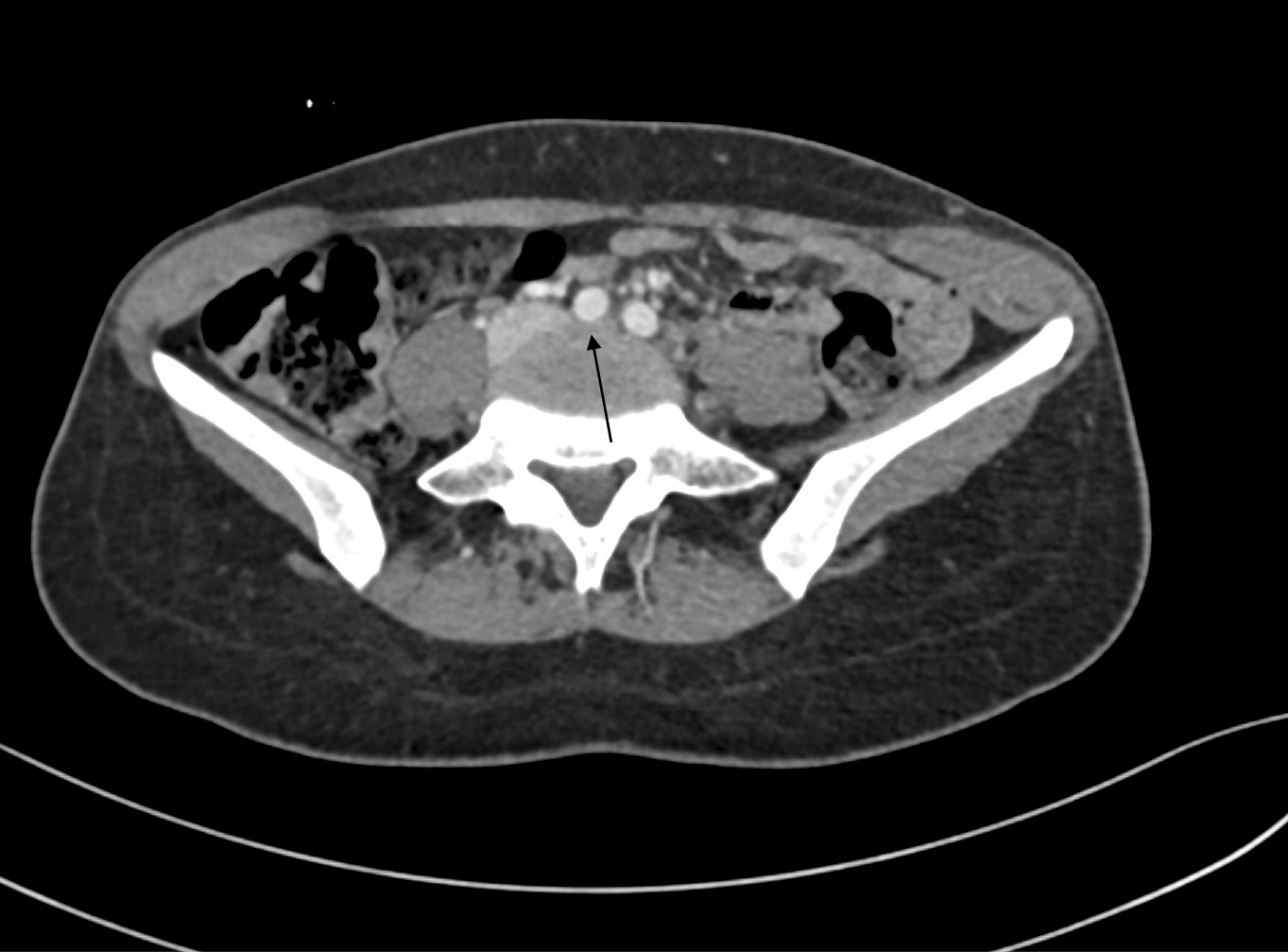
CT abdomen and pelvis with IV contrast (Venous Phase) at aortic bifurcation level, demonstrating the compression of the thrombosed Left common iliac vein (CIV) by the right CIA representing May Thurner Syndrome.
